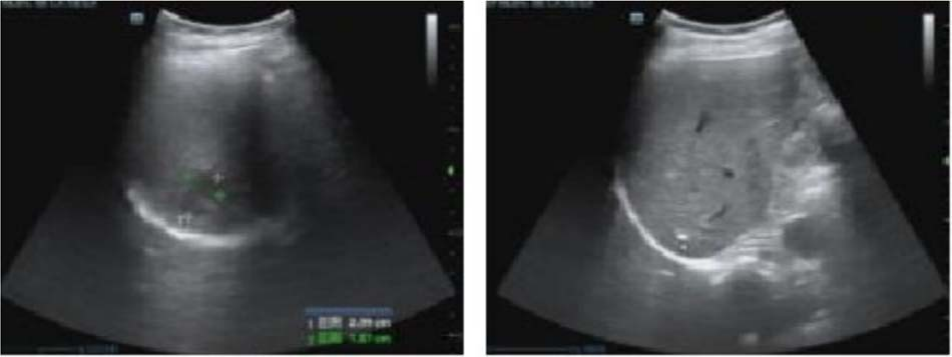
Abdominal color ultrasound findings on the 12th day after surgery.
